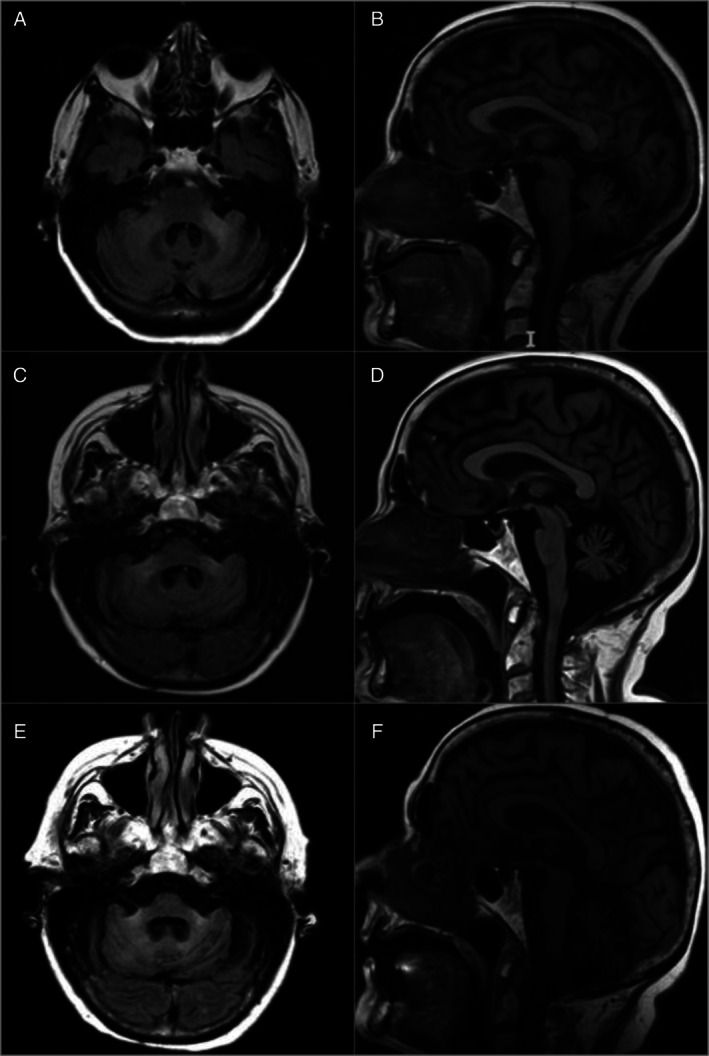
Serial MRI images taken over several years preceding the patient's acute presentation to the emergency room (ED). Note progressive atrophy and gliosis of the cerebellar peduncles and pons. (A, B) Four years prior to presentation. (C, D) Two years prior to presentation. (E, F) MRI from day of presentation to the ED.
